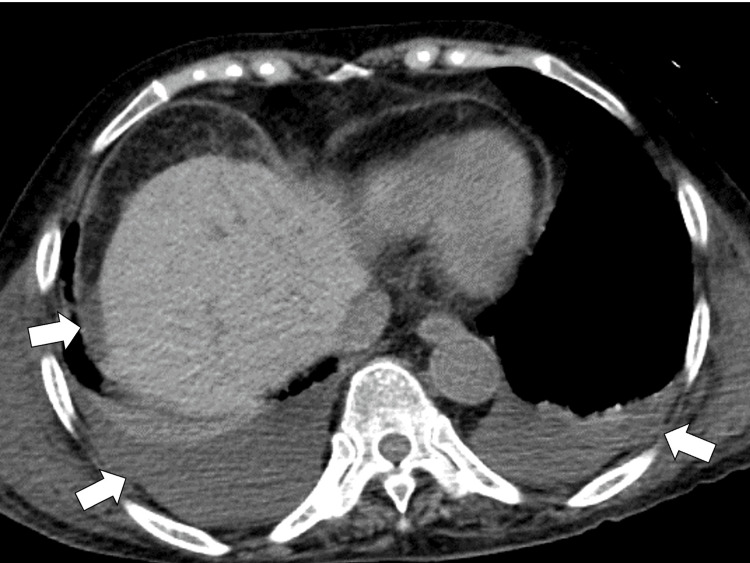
Neck-to-pelvic computed tomography clarifying bilateral pleural effusion and ascites (white arrows).
